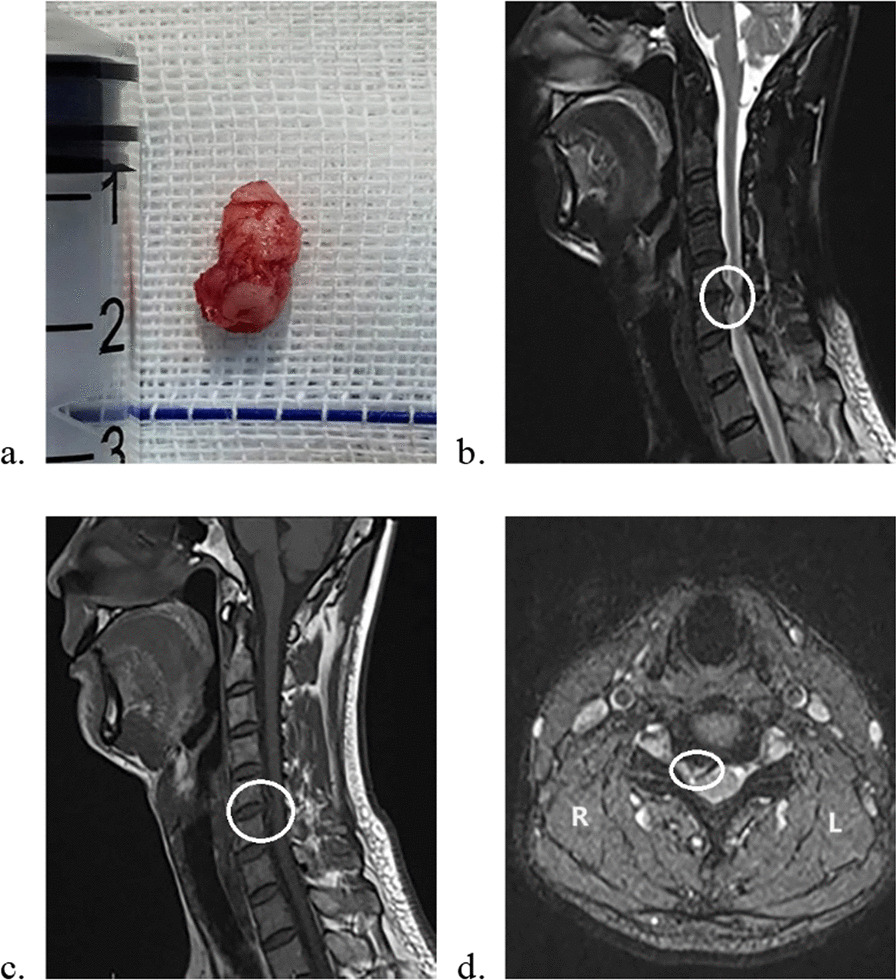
Sequestered disk in surgery. A 58-year-old woman complained of right-hand numbness adopted ACDF in our hospital. A sequestered disk was found in the anterior epidural space during the operation (a). The preoperative MRIs also showed an obvious cervical disk herniation at the C5-C6 level. *ACDF* anterior cervical decompression and fusion, *MRI* magnetic resonance imaging
